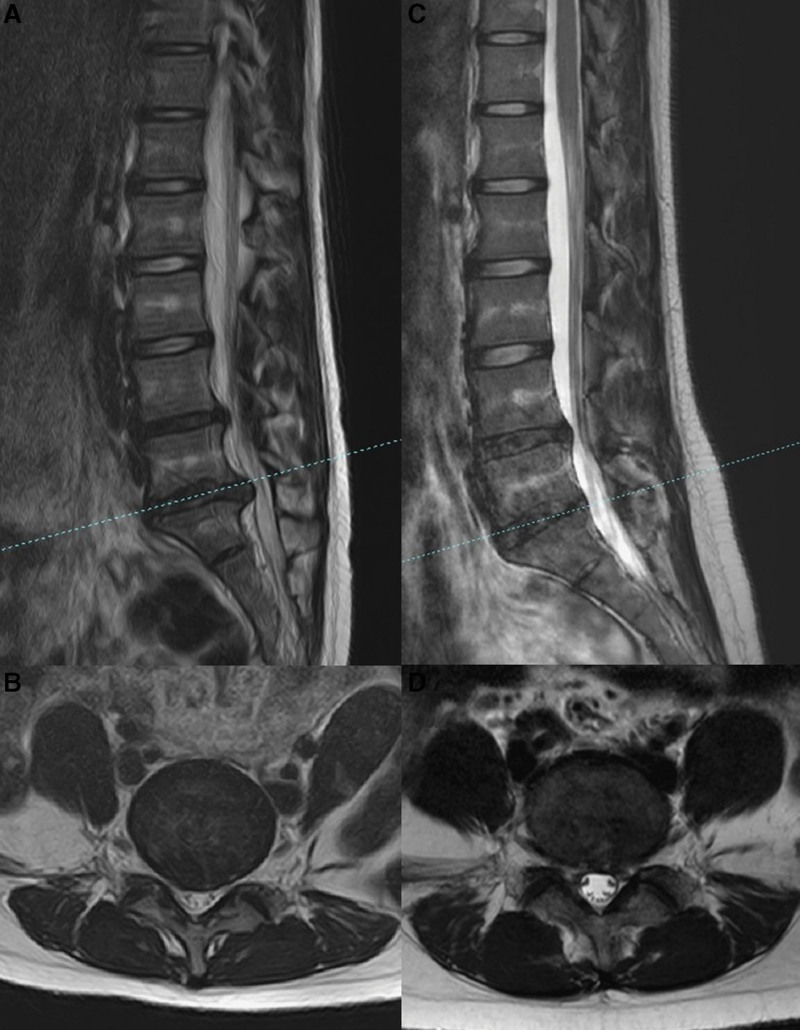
A 14-year-old girl presented with low back pain and an 8-month history of painful numbness radiating to the right lower limb. (a, b) Preoperative MRI showed disc herniation at the L4-5 and L5-S1 levels, especially at the L5-S1 level. (c, d) Postoperative MRI showed that the herniated disc had been removed, and both S1 roots were decompressed. MRI = magnetic resonance imaging.
